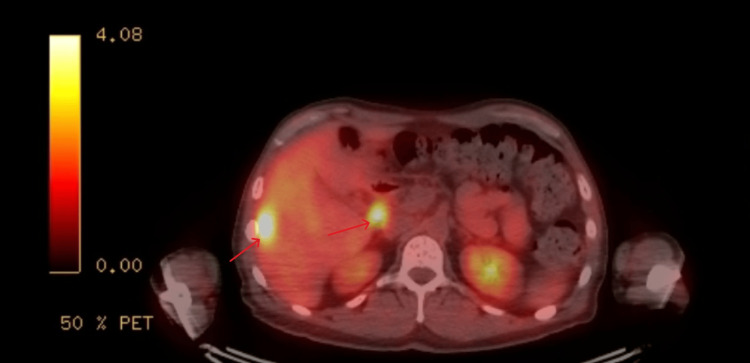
PET-CT showing multifocal, intensely FDG-avid lesions in the right hepatic lobe and an intensely FDG-avid 1.6 cm porta hepatis lymph node
